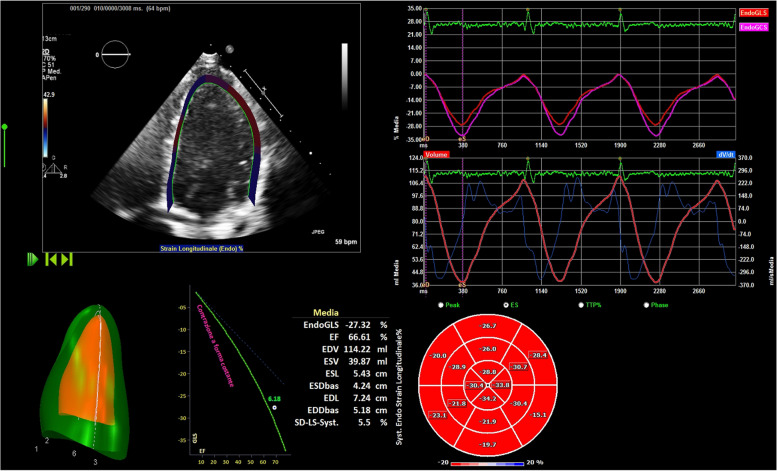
apical four-chamber view for Strain analysis
